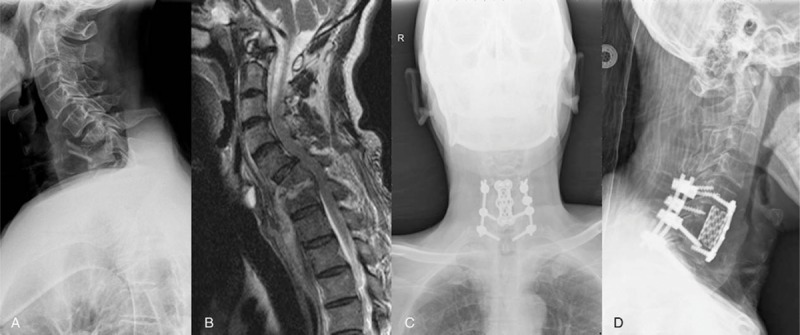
A 48-year-old man was diagnosed as having cervical tuberculous spondylitis (C6–7). X-ray, MRI showed the destruction of vertebral bodies of C6–7 (A–B). Final follow-up radiographs showed good bone fusion (C–D). MRI = magnetic resonance imaging.
